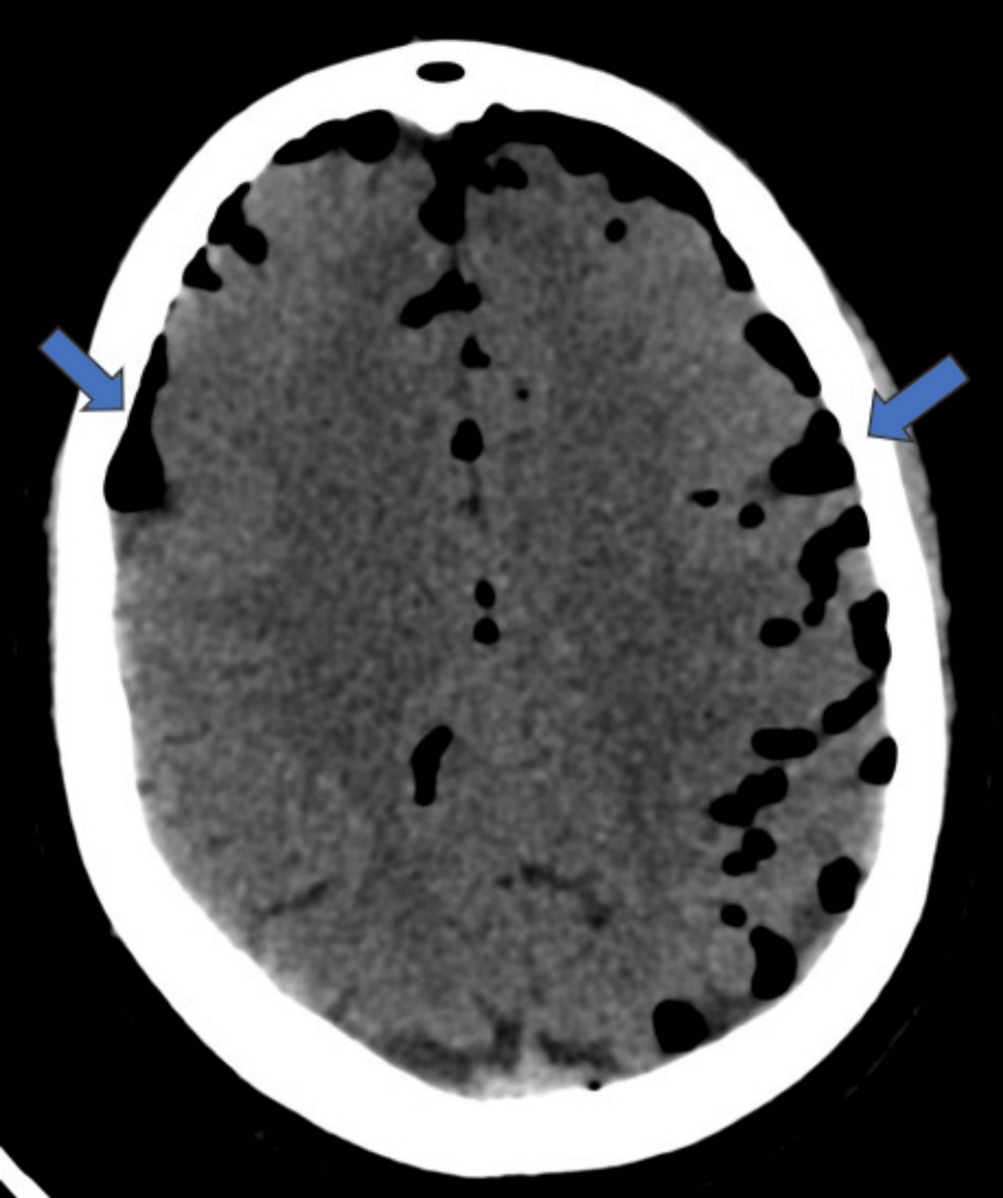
Axial CT brain at the level of frontoparietal lobes The image shows extensive air foci (blue arrows) along the subarachnoid and subdural spaces of bilateral cerebral convexities, predominantly on the left side.
